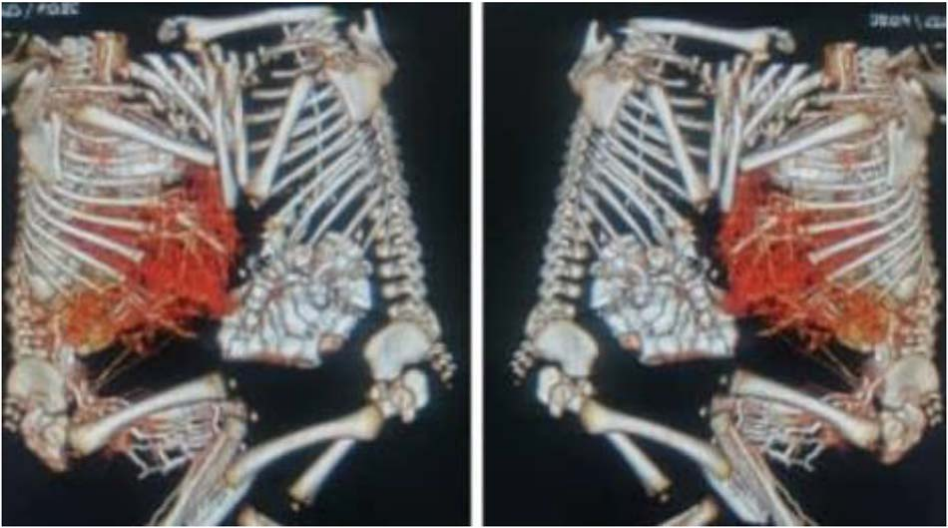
CT scan with contrast of case 2 showed omphalopagus type of conjoined twins with fusion of anterior aspect of the livers and separate other vital organs.
